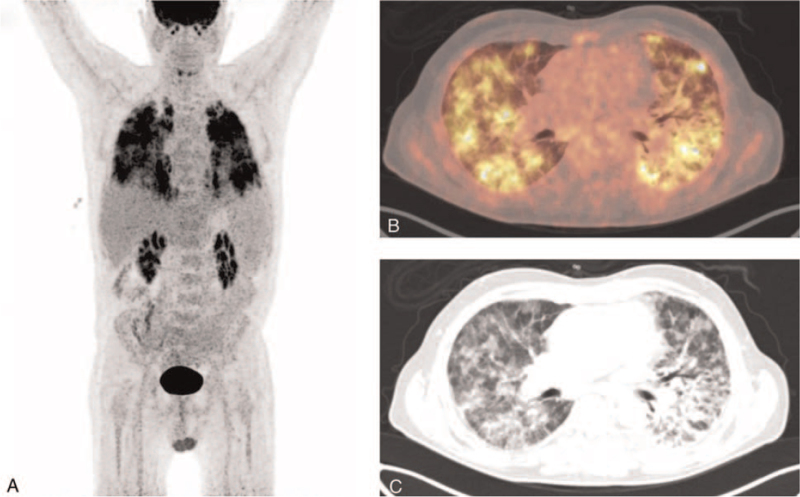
PET MIP (A), axial fused PET and contrast-enhanced CT images (B), and contrast-enhanced CT images. ^18^F-FDG = flourine-18 fluorodeoxyglucose, CT = computed tomography, PET = positron emission tomography.
